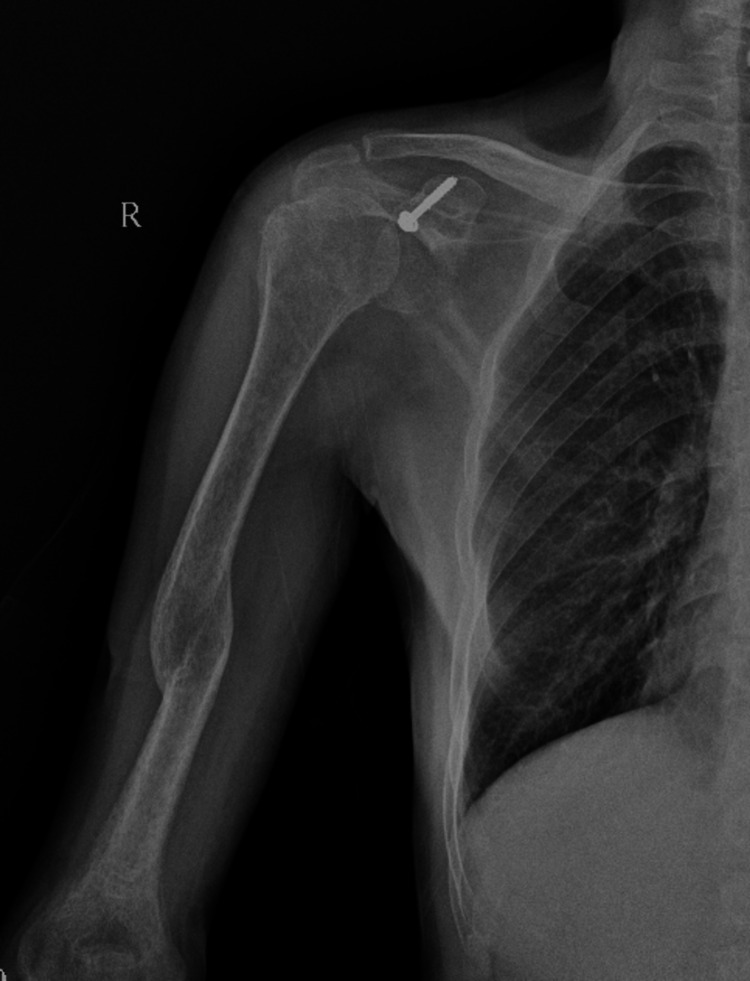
One-year follow-up X-ray showing well-reduced glenohumeral joint.
